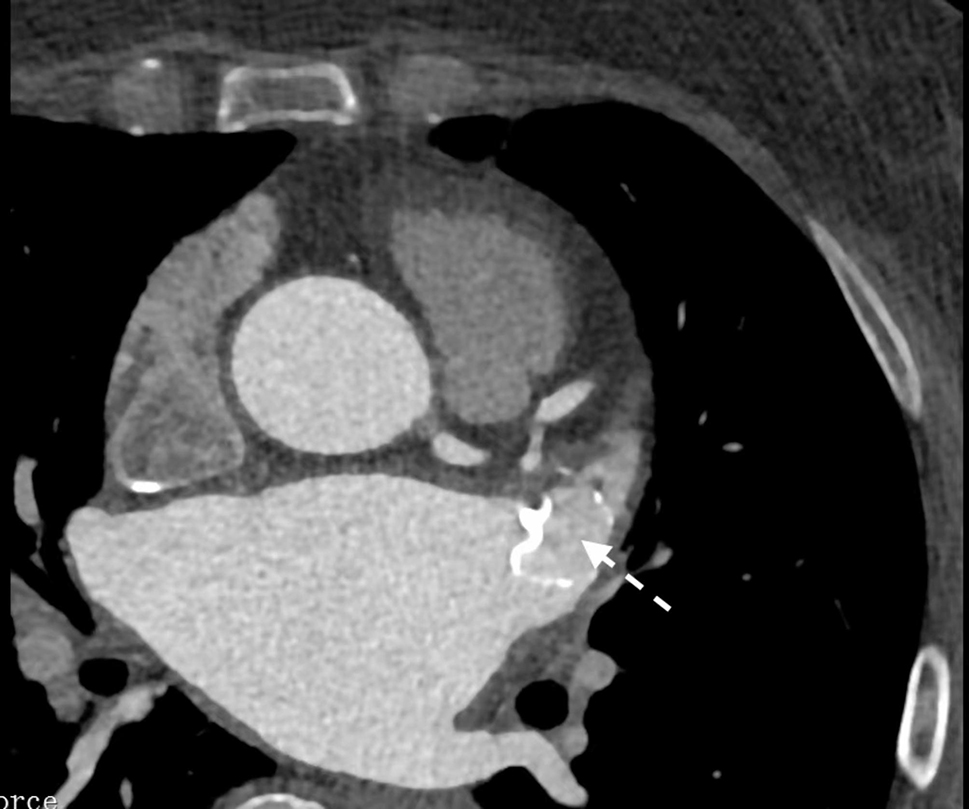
One case (a 60-year-old woman with persistent atrial fibrillation) for IDE at 6 weeks follow-up after LAAC. LAA CTA showed residual contrast agent flow inside the LAA (as shown by arrow)
